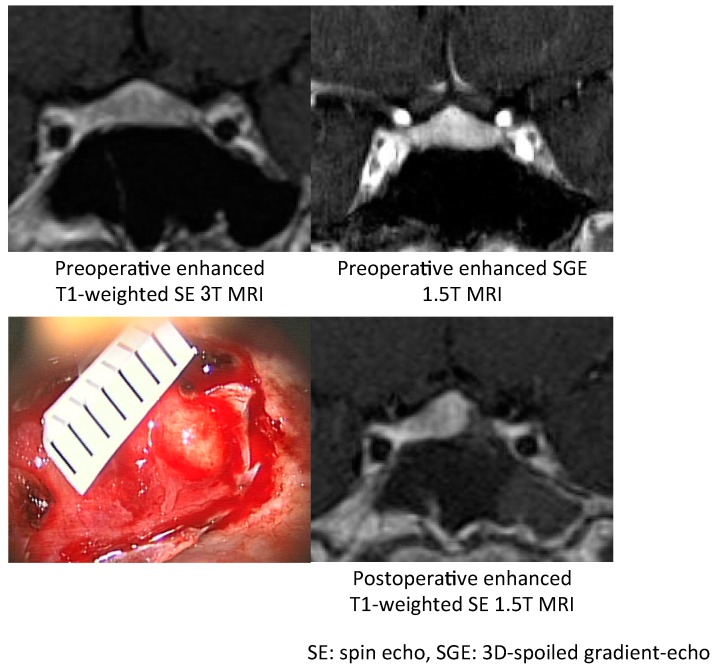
A patient of Cushing’s disease with negative-MRI^(151)^. Extensive MRI studies including 3T MRI failed to demonstrate any findings suggesting tumor but inferior petrosal sinus sampling (IPSS) suggested pituitary origin of ACTH-dependent CD in a 20-year-old woman. A 3-mm diameter micro-tumor was found on the left side of the pituitary as predicted by IPSS. Selective tumorectomy resulted in complete remission of the disease. No abnormal findings were found in preoperative MRI even after review in MRI scans retrospectively comparing them to postoperative MRI findings.
